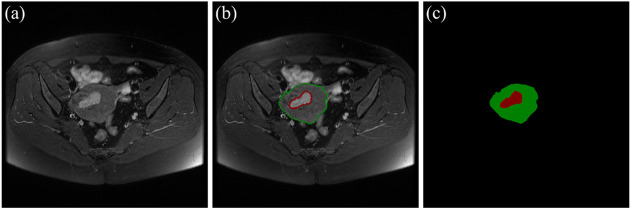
(A) The original MRI image. (B) The original MRI image with ground-truth contours of the uterus and tumor, where the red outline is the ground-truth of the tumor and the green outline is the ground-truth of the uterus. (C) The label image that LabelMe transforms from the ground-truth contours in (B) which is used for DL model training.
